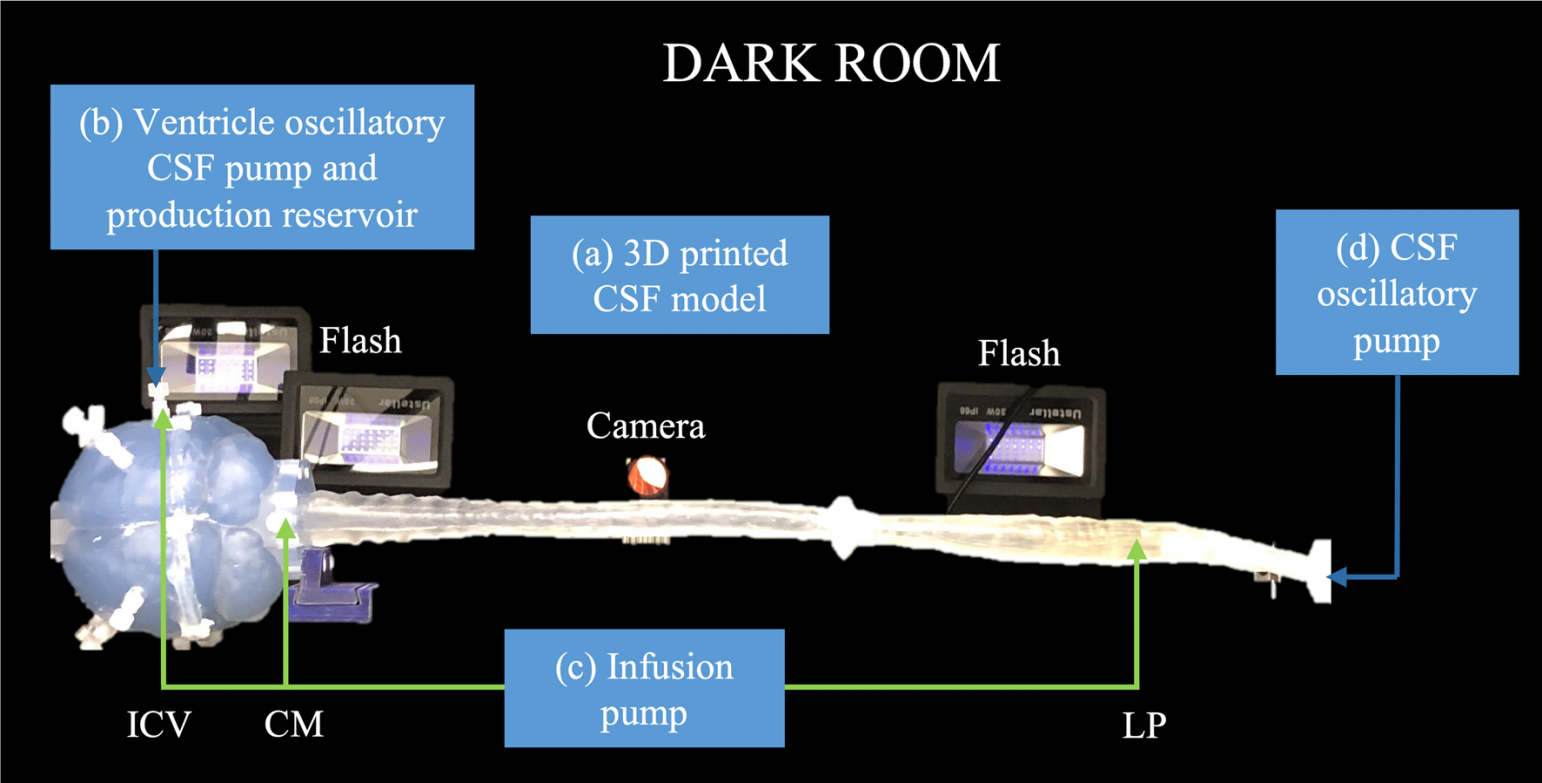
Overview of in vitro system and bench top experiment setup a 3D printed n-vitro model of the CSF system with placement of flash lightning and imaging camera. b CSF reservoir tank and custom ventricular oscillatory CSF flow pump in the ventricles. c Continuous injection pump for tracer injection at ICV, CM, and LP. d Oscillatory pump to induce CSF oscillations in the SAS to match the CSF flow field acquired by phase contrast-MRI
